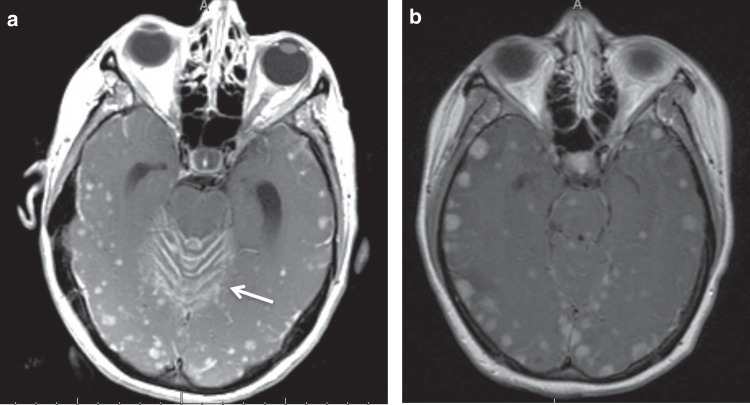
Patients with typical miliary metastatic lesions seen on neuroimaging. a Example of miliary metastasis with concomitant imaging diagnosis of leptomeningeal disease (LMD denoted by arrow). b Example of miliary metastasis with lesions of various sizes, reinforcing our decision to include lesion number and anatomical distribution in our radiographic definition but not rely on lesion size.
